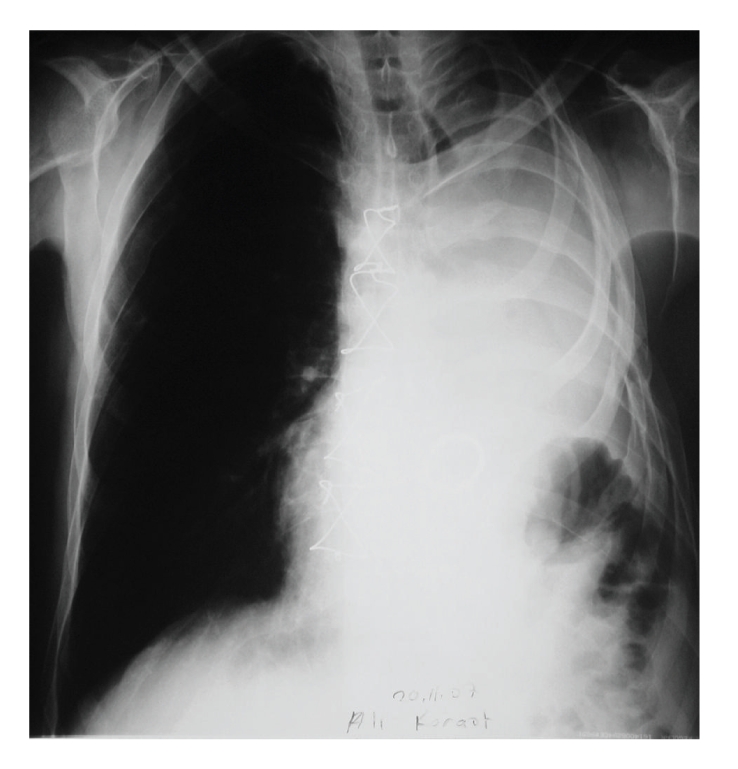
Chest roentgenogram of the patient postoperatively. Note the hyperinflated right lung with leftward displacement of the mediastinal structures.
